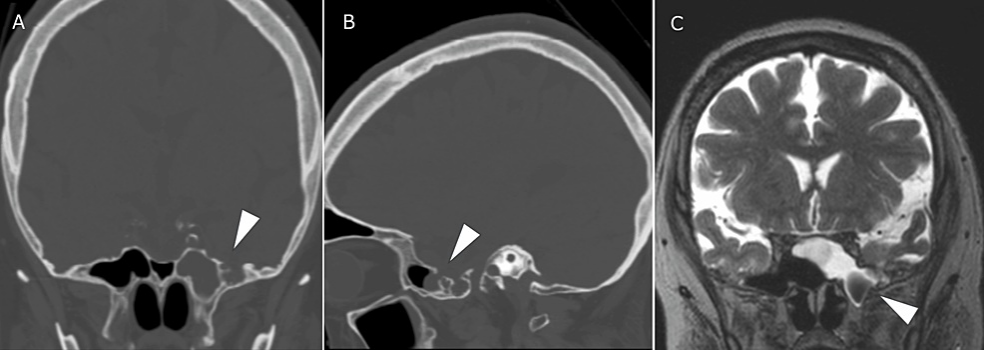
The CT and MRI scans show meningoencephalocele protruding into the left sphenoid sinus through the bone defect and CSF in the sphenoid sinus. Head CT showing a bone defect (arrowheads, A and B) in the left middle cranial fossa communicating with the sphenoid sinus. MRI showing meningoencephalocele in the sphenoid sinus (arrowhead, C) and accumulated cerebrospinal fluid within the sphenoid sinus. CT: computed tomography; MRI: magnetic resonance imaging; CSF: cerebrospinal fluid
